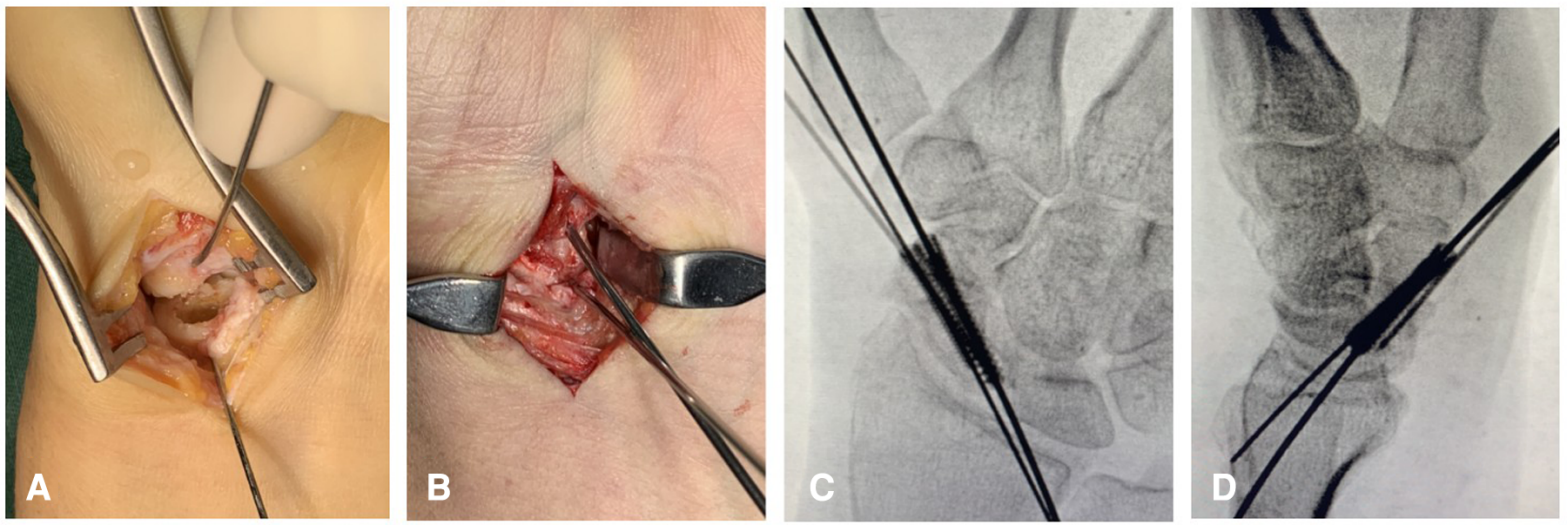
Fibrous tissue fragments were thoroughly debrided, leaving a shell of intact cartilage (A). Cancellous graft obtained from the patients’ iliac crest was impacted into the shell, and a wedge-shaped corticocancellous graft was inserted into the defect to maintain the reduction. Kirschner wires were used as joysticks to distract across the nonunion site (B). Two cannulated screws were inserted into the scaphoid along the guide wires, and the length and position of the screws were confirmed by x-ray (C, D).
